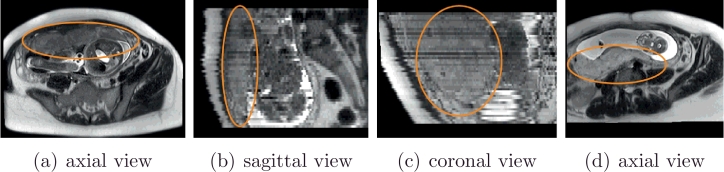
Examples of fetal MRI. (a), (b) and (c) are from one patient while (d) is from another. Note the motions and different appearance between slices in (b) and (c). The placenta is anterior in (a), but posterior in (d).
